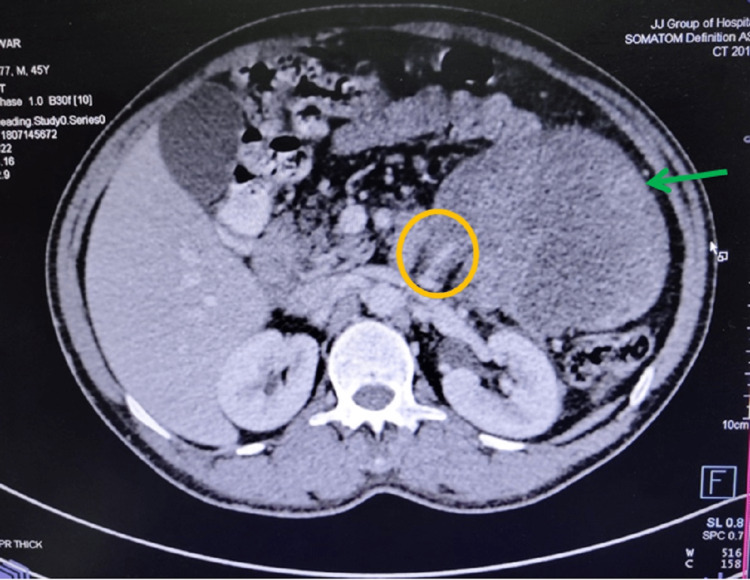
Axial section of CT abdomen showing the tumour with feeding vessel and its relation with the surrounding structures Green arrow - Heterogeneously enhancing, solid lesion Yellow circle - Feeding vessels
